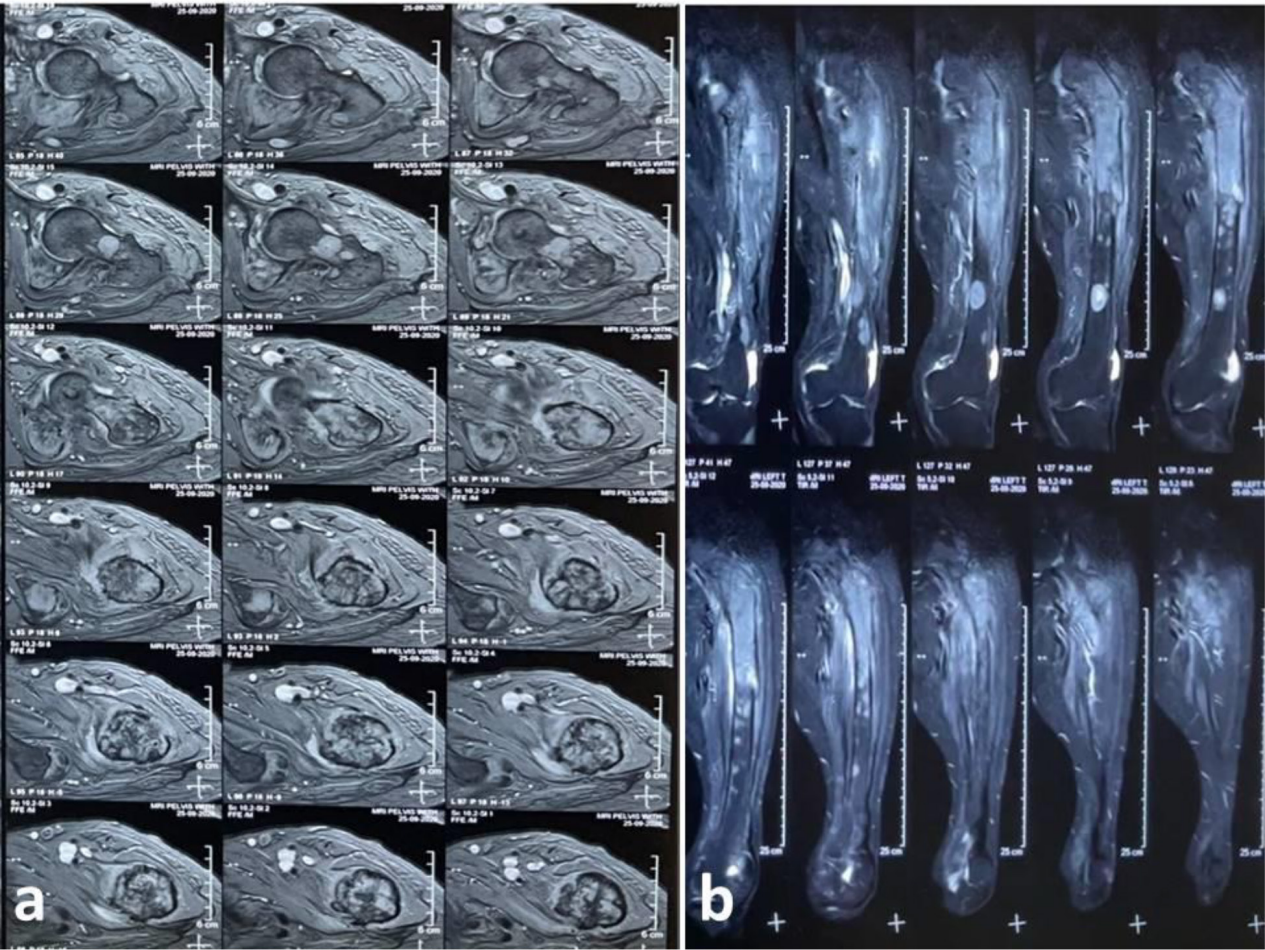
Magnetic resonance T1-weighted axial images of the proximal left femur show multiple hypodense lesions in the neck, intertrochanteric, and proximal femur (a), and T2-weighted coronal images of the left thigh showed perosseous edema and edema of the adjacent muscles. Posterior and medial cortexes are eroded, and soft tissue within the bone extends into the adjacent perosseous plane (b).
